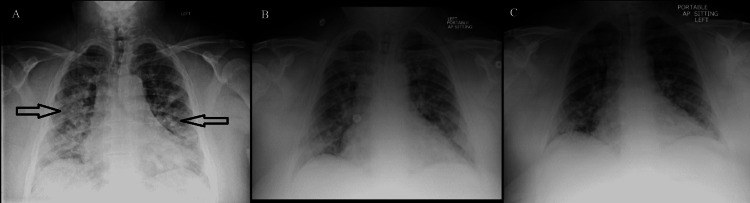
Twin B: chest X-ray on day one (A), day three (B), and day five (C). Arrows depict diffuse bilateral infiltrates.
